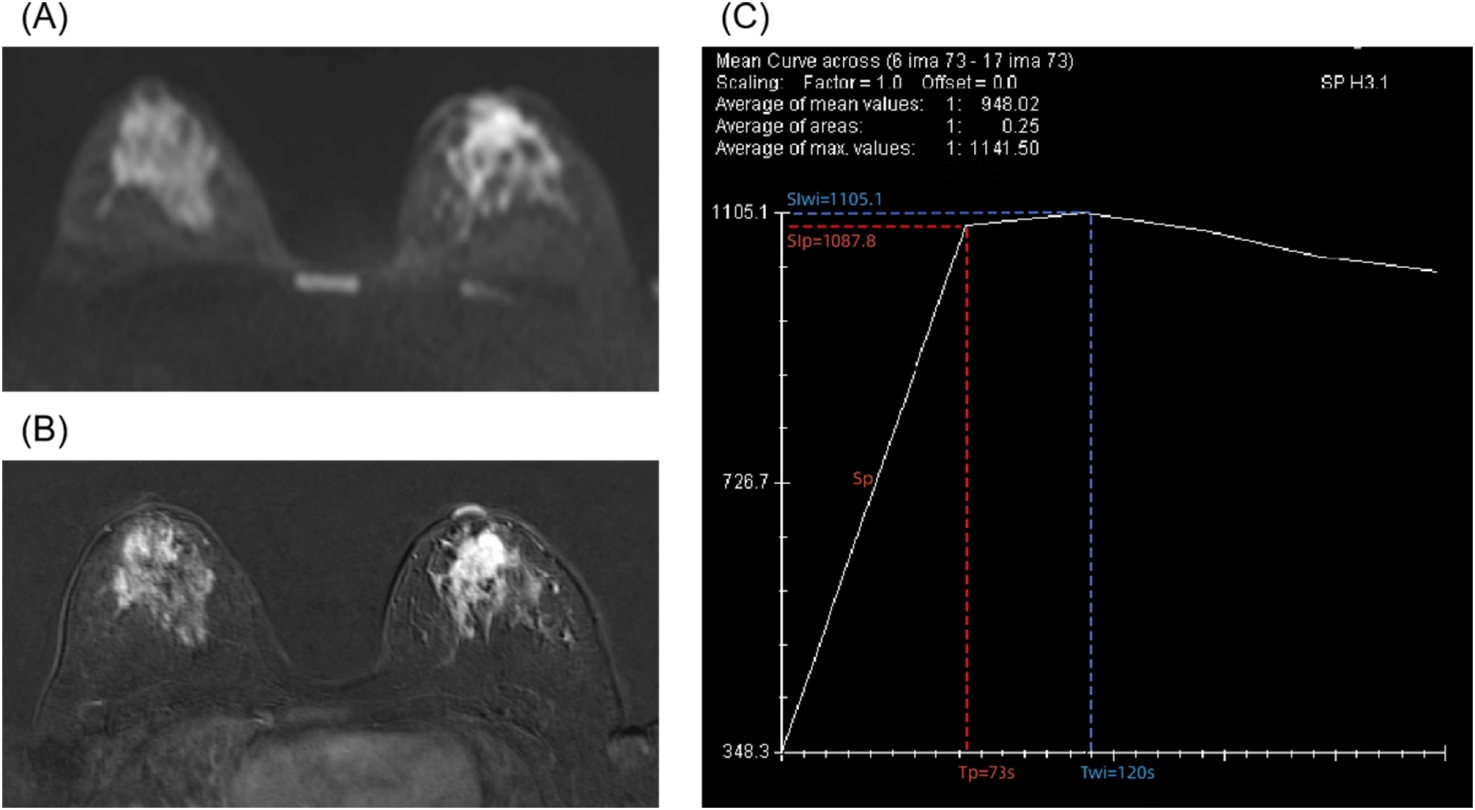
A 28-year-old female patient with grade III invasive ductal carcinoma of the left breast. (A) Diffusion-weighted imaging shows a high signal mass with an apparent diffusion coefficient (ADC) of 0.9 × 10^−3^ mm^2^/s. (B) Enhanced T1-weighted imaging shows irregular, non-enhanced necrotic area with lobulated signs at the margins. (C) A type II time–intensity curve (TIC) is shown. The semi-quantitative parameters are signal intensity of pre-contrast (SIpre) = 348.3, time to peak (Tp) = 73 s, peak signal intensity (SIp) = 1,087.8, slope of peak (Sp) = 10.13, enhancement rate of peak (ERp) = 2.12, time of wash-in (Twi) = 120 s, signal intensity of wash-in (SIwi) = 1,105.1, slope of wash-in (Swi) = 6.31, and enhancement rate of wash-in (ERwi) = 2.17.
